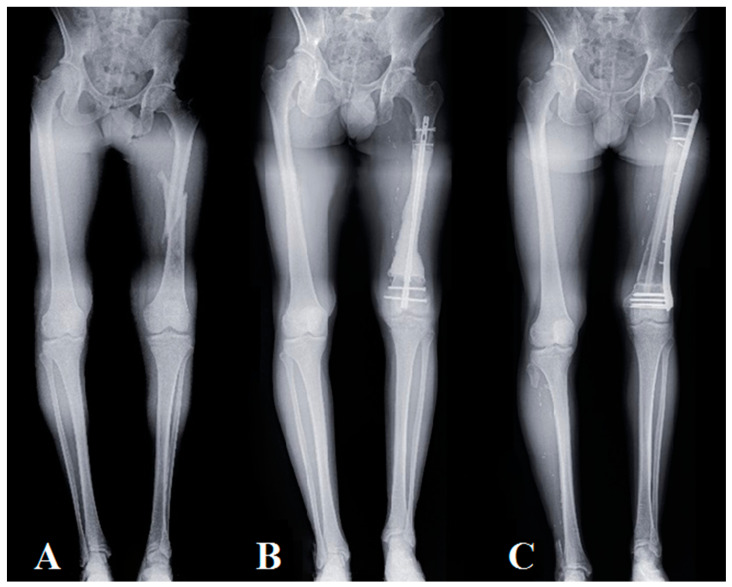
Progression of a Capasquelet technique reconstruction, using a pangonogram X-ray view, in a 28 year old patient with high-grade osteosarcoma (patient No. 2): (A) preoperative X-ray, fractured high-grade osteosarcoma with a length inequality of 80 mm; (B) postoperative X-ray of the 1st surgical stage after carcinologic resection and placement of the spacer on an intramedullary nail with a length inequality of 60 mm (post-T1 delay: 1 month); (C) postoperative X-ray at 1 year, residual lower limb inequality of 40 mm.
